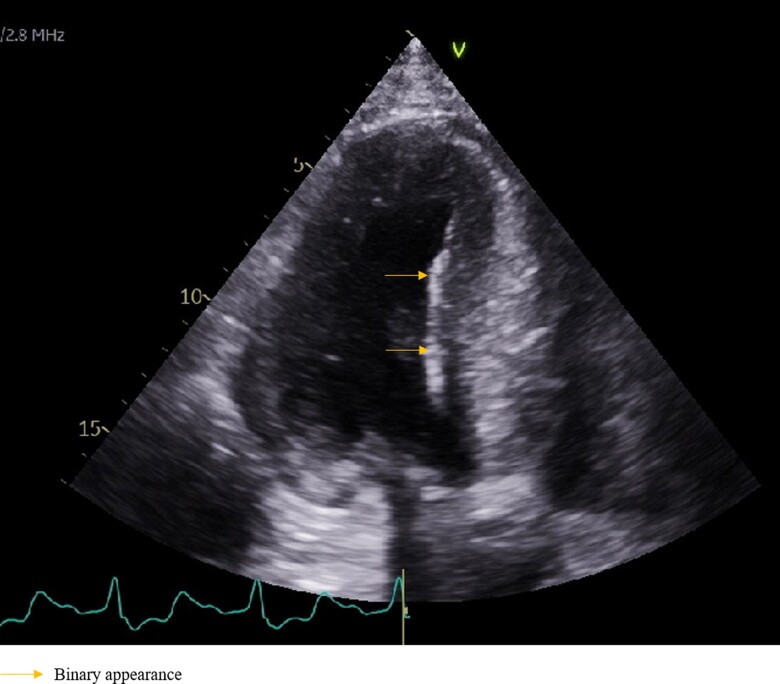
Patient 3 echocardiogram showing binary appearance of the myocardium.
